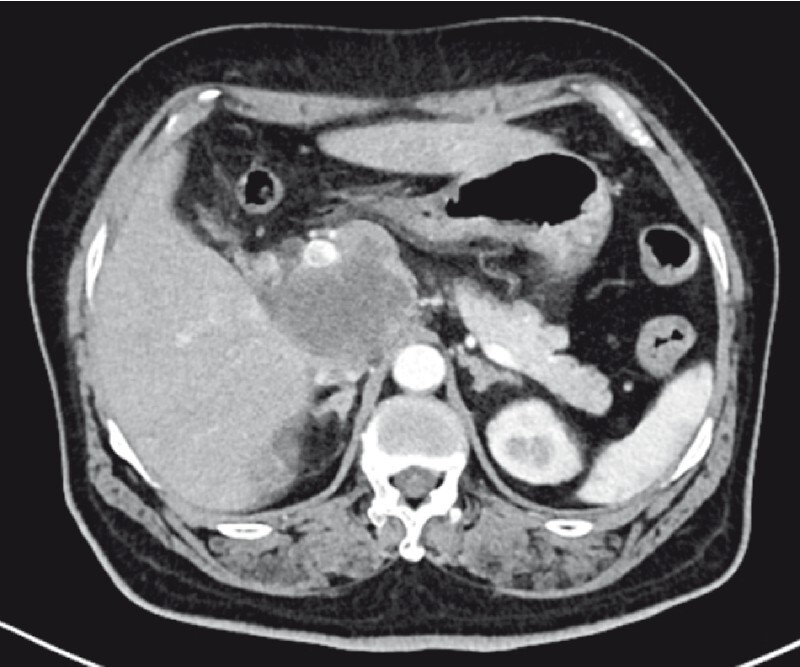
Computed tomography revealed a mass in the head of the pancreas.
